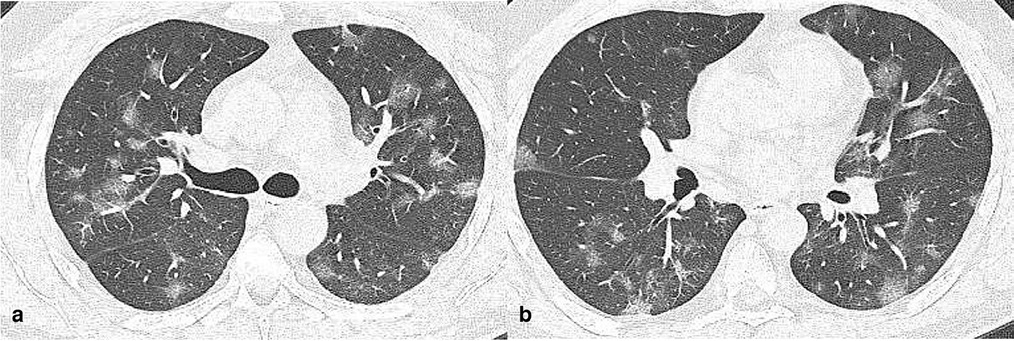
Axial CT images (a, b) of a 34-year-old male infected by SARS-CoV-2 α variant (3 days since symptoms onset). CT showed centrally and peripherally located multifocal GGO opacities with mainly involvement of upper lobes.
